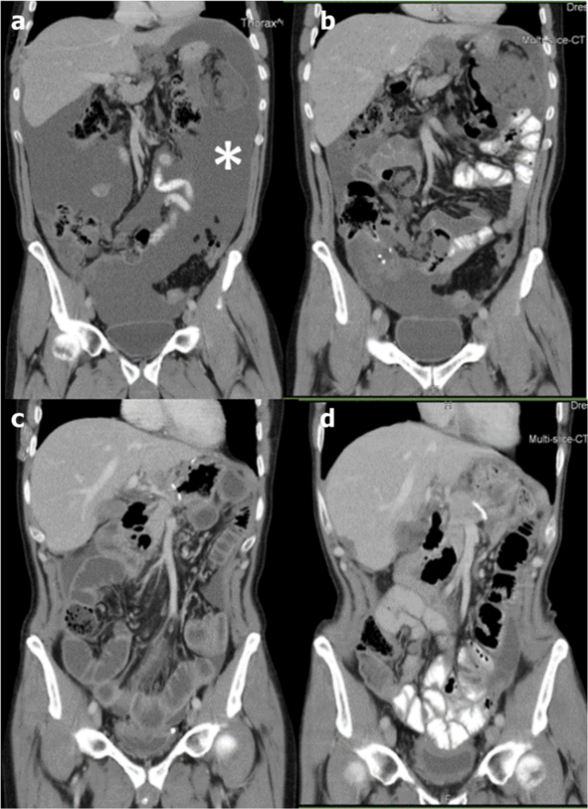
Contrast-enhanced CT scans of a 57-year-old male patient with peritoneal metastasis of an appendiceal cancer. a Image after 12 cycles of combination palliative chemotherapy with FOLFOX4 and 6 cycles of FOLFIRI showing active disease with massive ascites (*asterisk*). b Evolution after 5 cycles of PIPAC with low-dose cisplatin and doxorubicin showing partial tumor response according to RECIST 1.1 criteria, in particular ascites control. c Postoperative image after cytoreductive surgery and hyperthermic intraperitoneal chemotherapy (HIPEC). d CT scan 6 months after CRS and HIPEC showing beginning recurrence (minimal ascites and tumor node on the lateral liver surface). The patient survived 46 months after diagnosis, 25 months after first PIPAC, and 18 months after CRS and HIPEC
